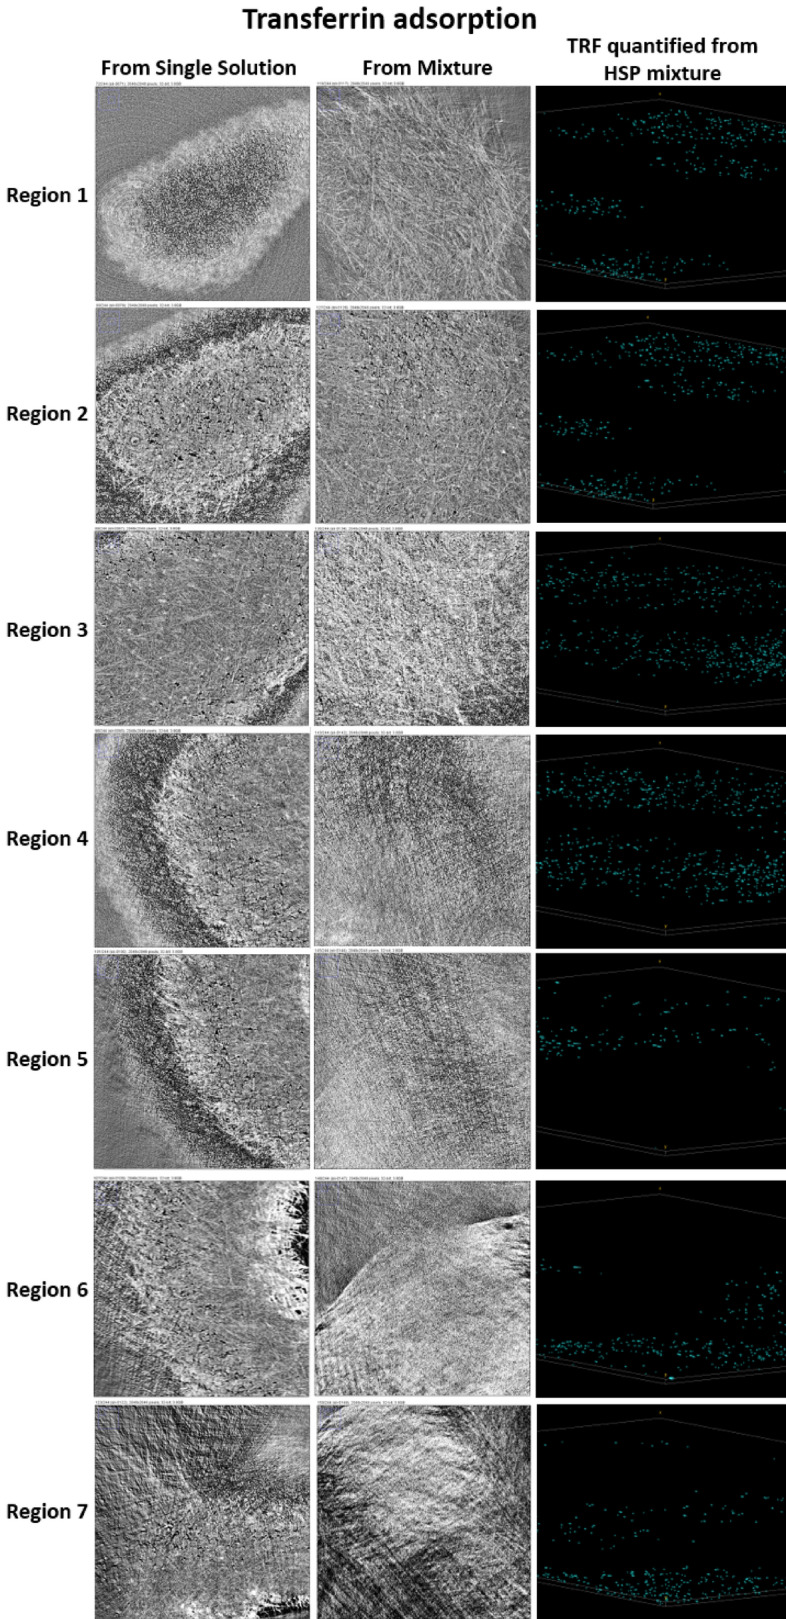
Converted radiographs of PES membrane with adsorbed FB from its single solution and HSP, and visualization of the FB distribution across the membrane thickness.
